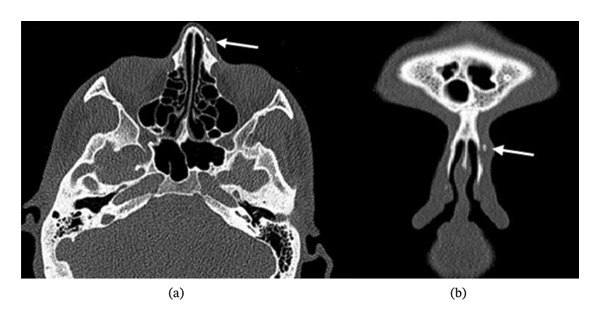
(a) Paranasal CT, axial imaging plane. (b) Paranasal CT, coronal imaging plane (white arrow indicates the bone spicule).
